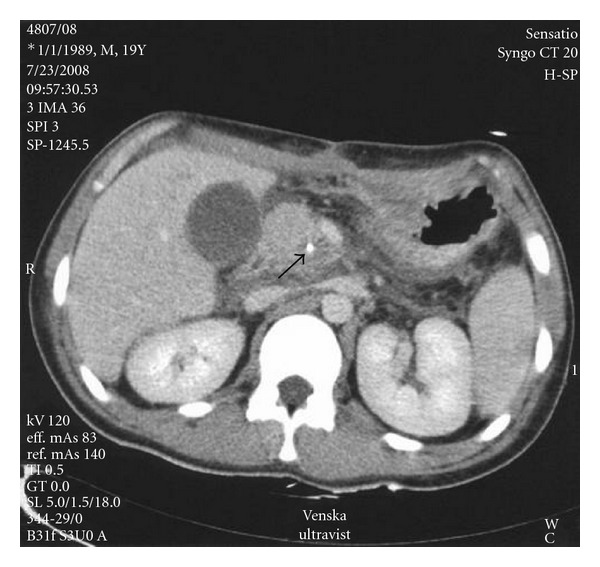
CT scan 24 hours post embolisation in delayed venous phase (45 sec), collimation 5 mm showing complete thrombosis of pseudoaneurysm. Hyperdense small round object (arrow) consistent with coils.
